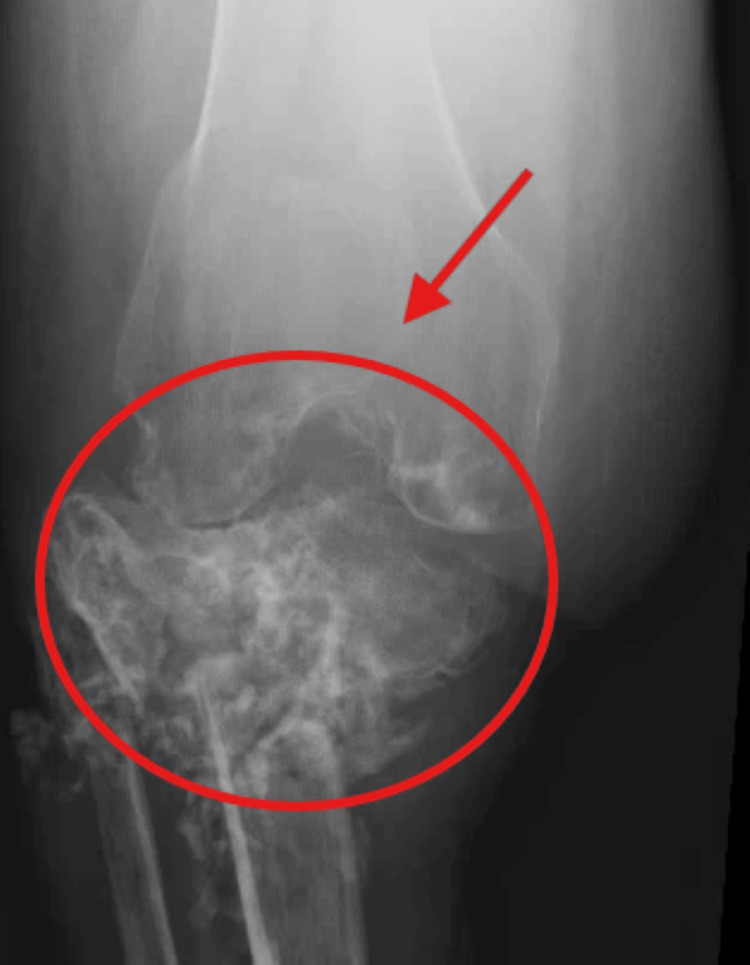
Plain radiograph (lateral view) of the left knee and tibia/fibula (current)
